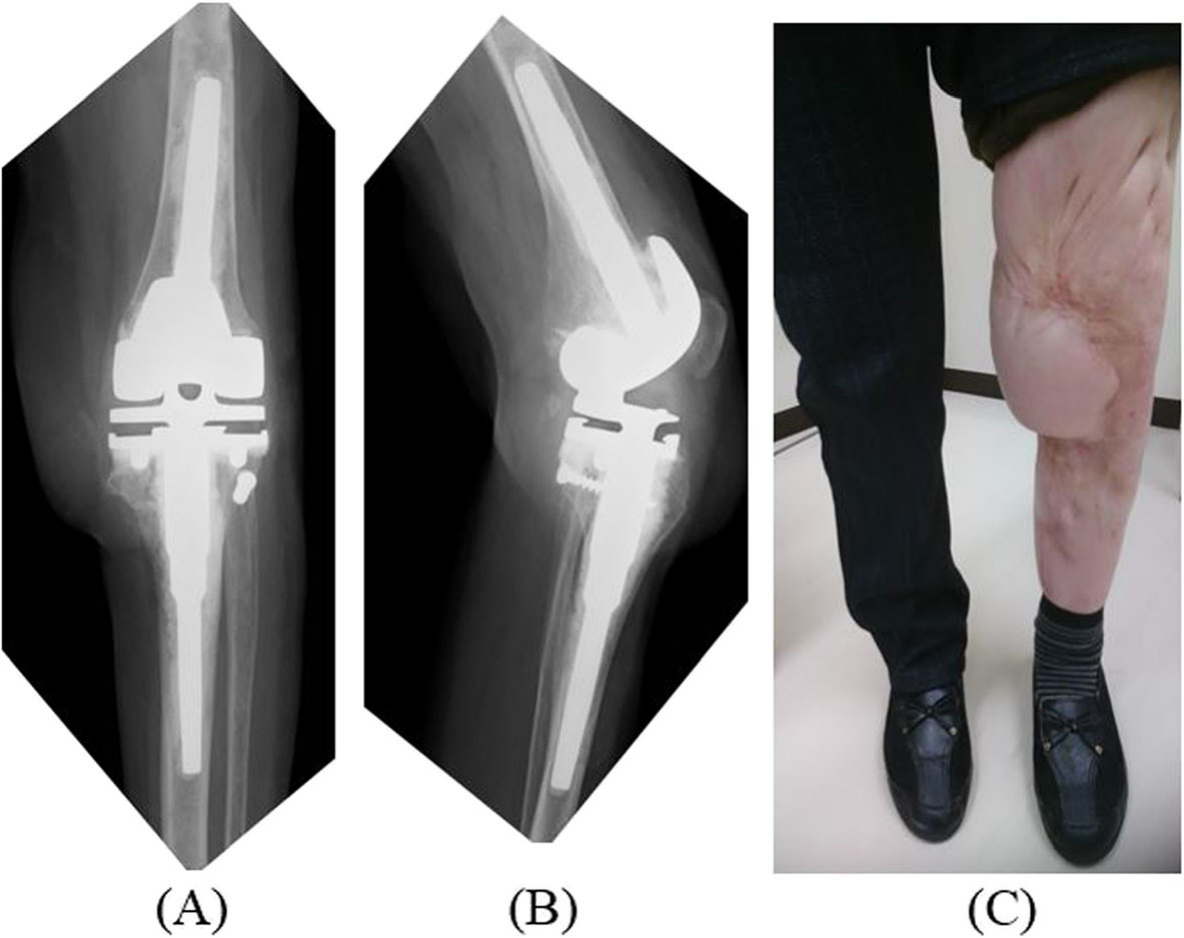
Postoperative X-ray and clinical photograph. a Six years postoperative antero-posterior X-ray. b Six years postoperative medio-lateral X-ray. c Six years postoperative clinical photograph
